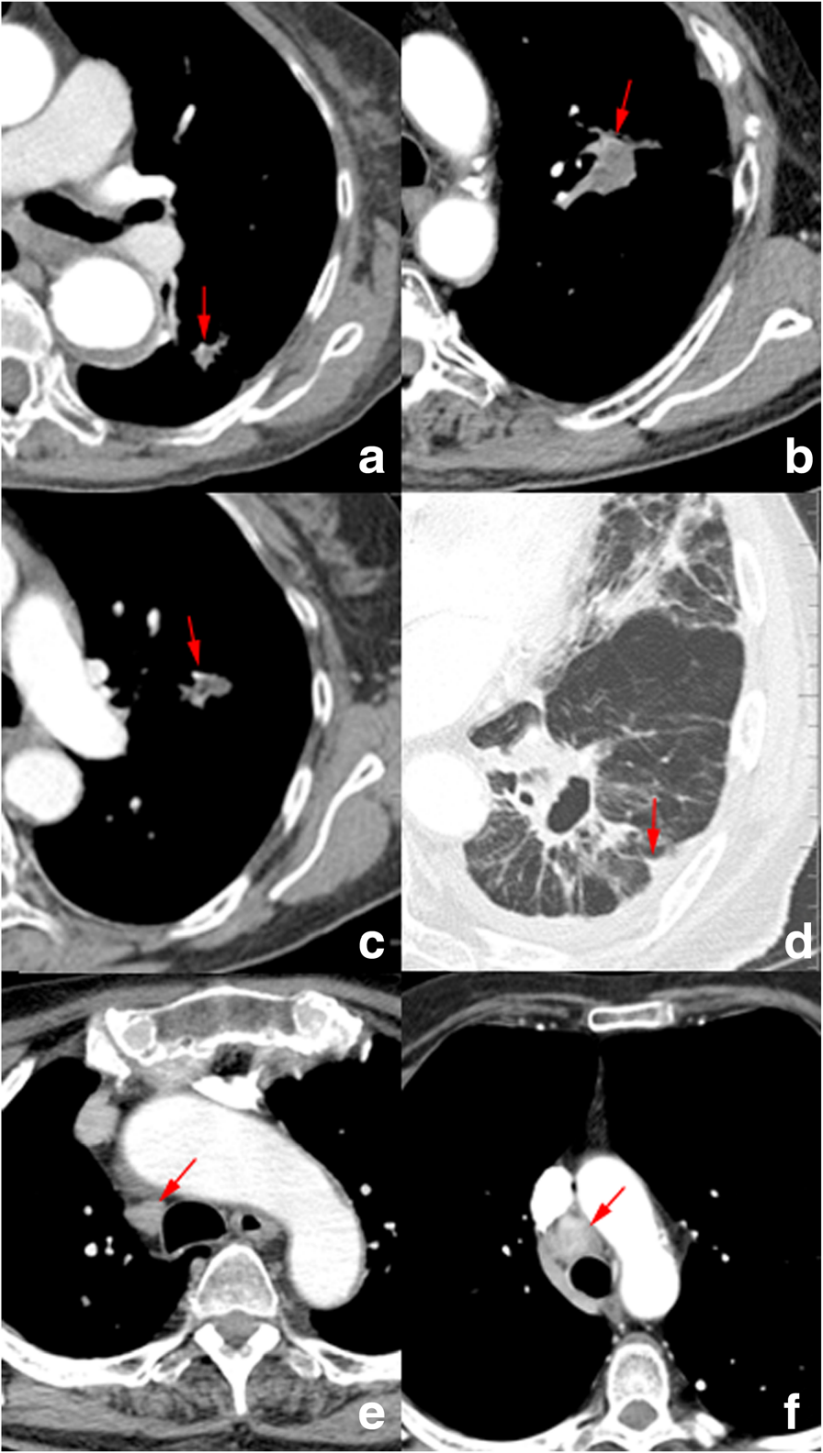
Examples of CT images showing typical semantic features (a - b: vascular involvement; c: vessel attachment; d: pleural retraction: e: benign lymphadenopathy, f: malignant lymphadenopathy)
